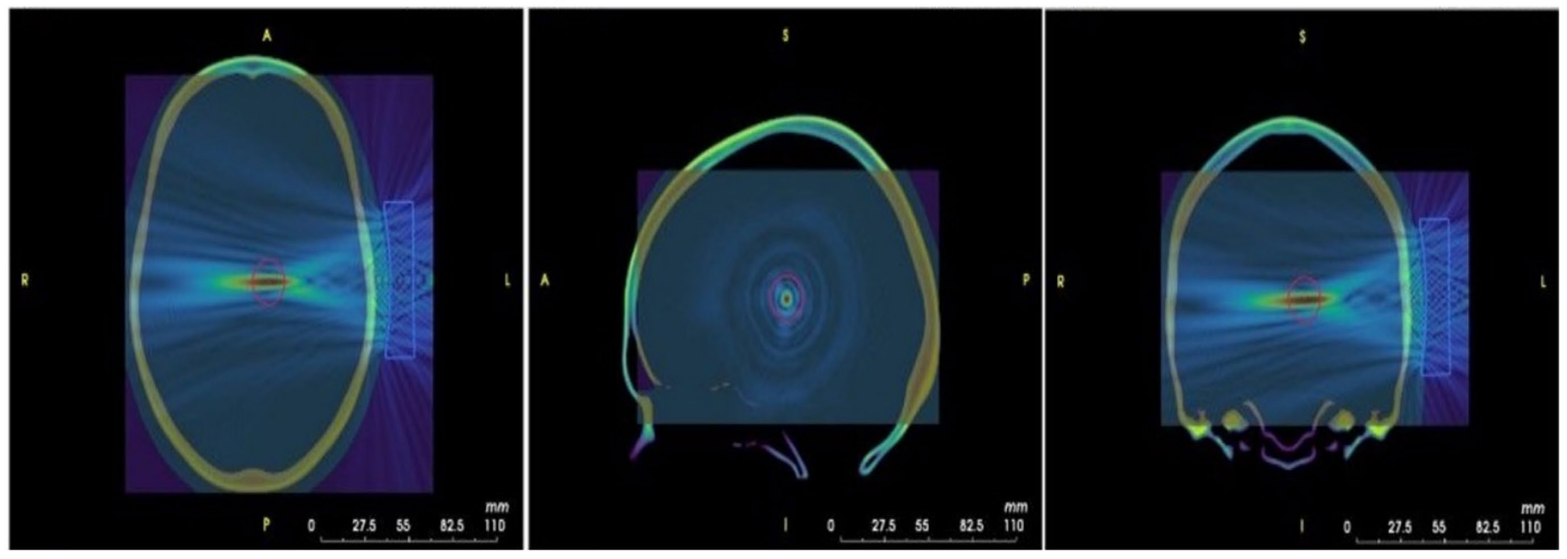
Pressure maps derived from ultrasound modeling superimposed on the MRI image of the MNI template, which was obtained from k-Plan.
